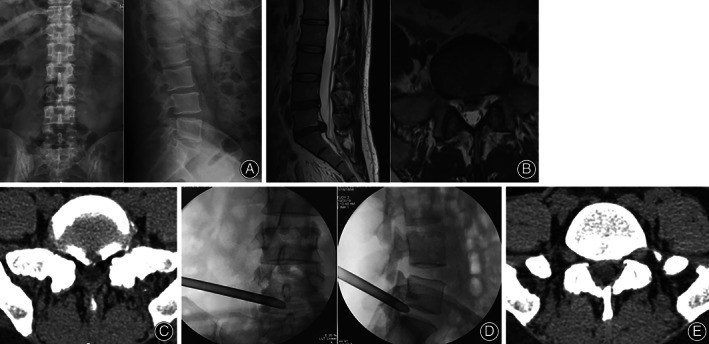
Woman, 45 years old. Main complaint: lateral pain of right leg with numbness for half a year. (A) Preoperative X‐ray films. (B) Preoperative MRI shows that L_5_S_1_ disc herniation and the protruding nucleus pulposus compresses the dural sac. (C) Preoperative CT image shows L_5_S_1_ disc herniation. (D) The position of the working cannula using the intervertebral foramen approach. (E) CT image shows that the nucleus pulposus was completely removed.
